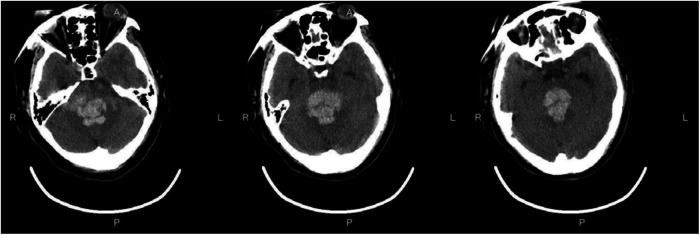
Preoperative imaging: Figure 3 shows a representative preoperative CT scan of a patient with PHBH.
